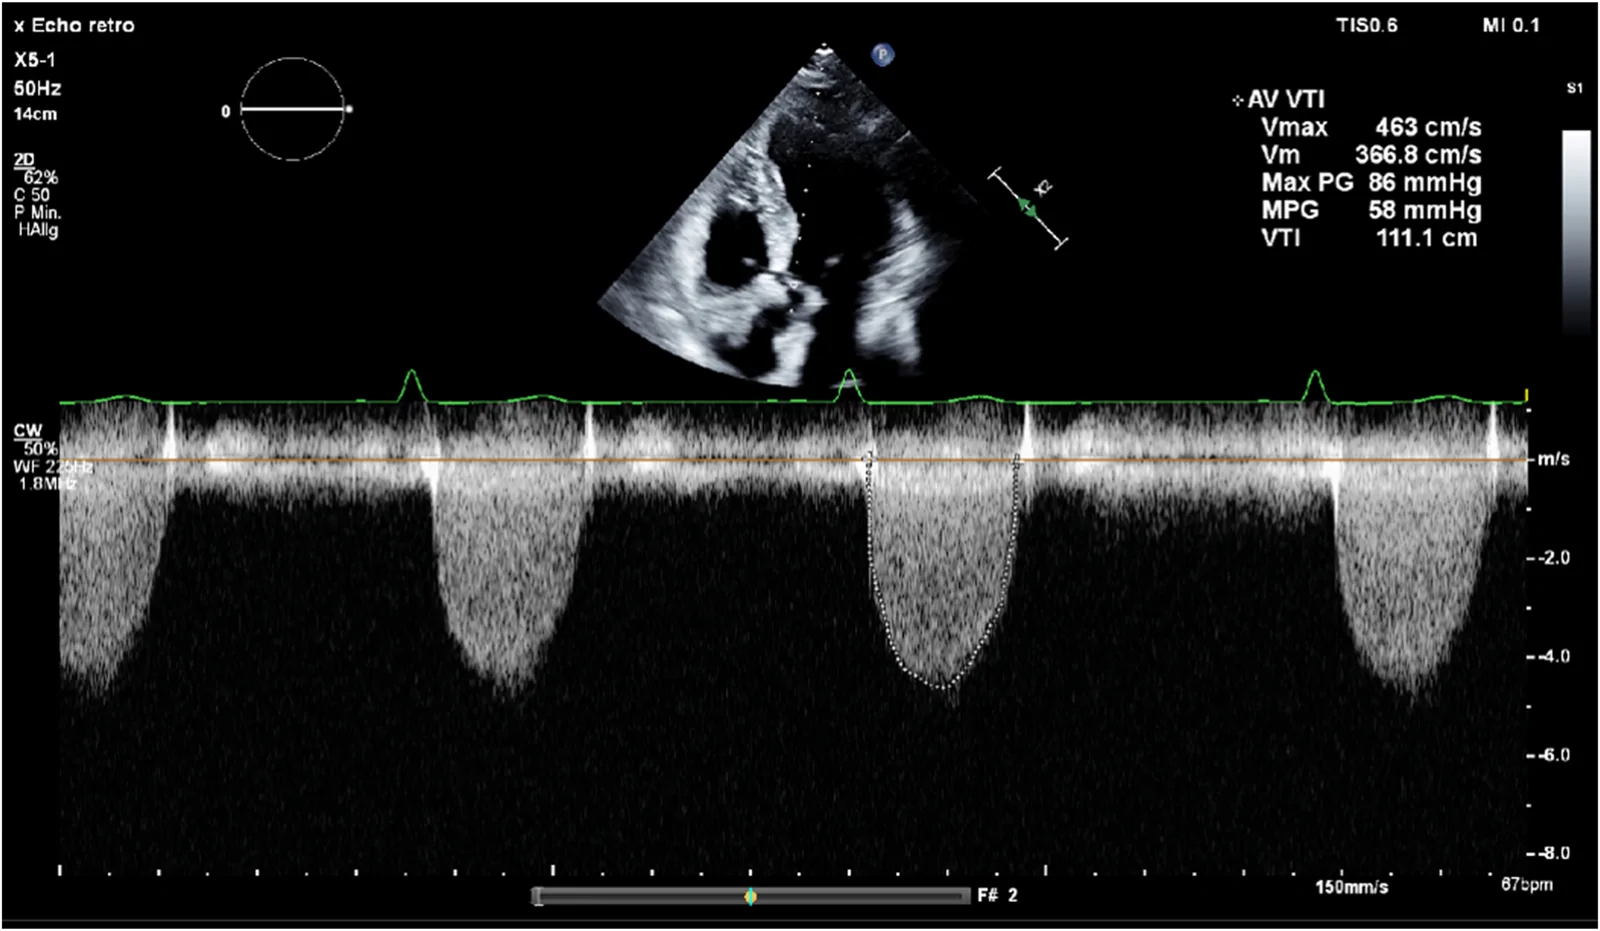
Continuous-Wave Doppler Assessment of Severe Bicuspid Aortic Stenosis Transthoracic echocardiography in an apical 5-chamber view with focused visualization of the left ventricular outflow tract and aortic valve (AV). Continuous-wave Doppler demonstrates markedly elevated systolic velocities consistent with severe aortic stenosis (peak velocity 4.63 m/s; peak gradient 86 mm Hg; mean gradient 58 mm Hg). MPG = mean pressure gradient; VTI = velocity time integral.
